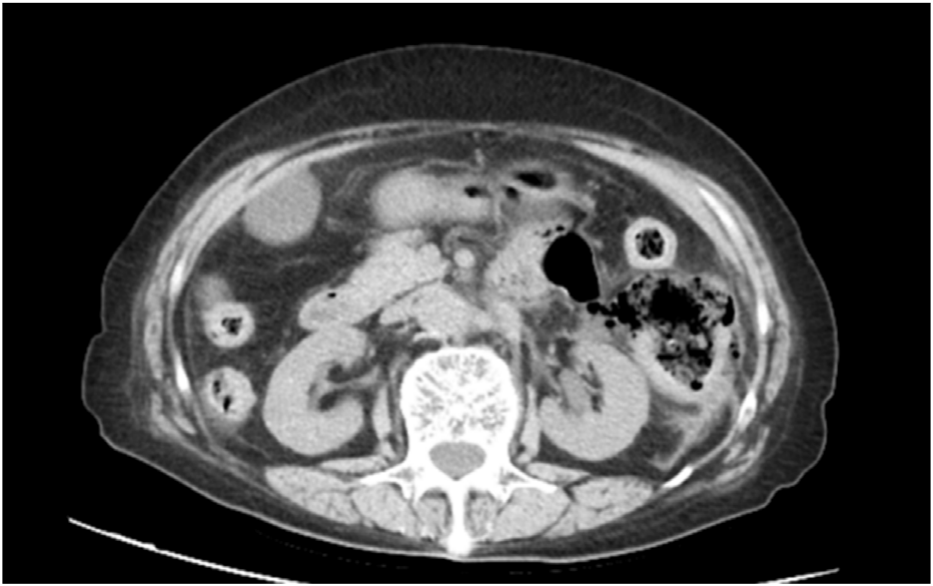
Abdominal computed tomography (CT) revealed mild thickening of the descending colon wall accompanied by free gas and surrounding exudation, a perforation of the digestive tract was considered.
